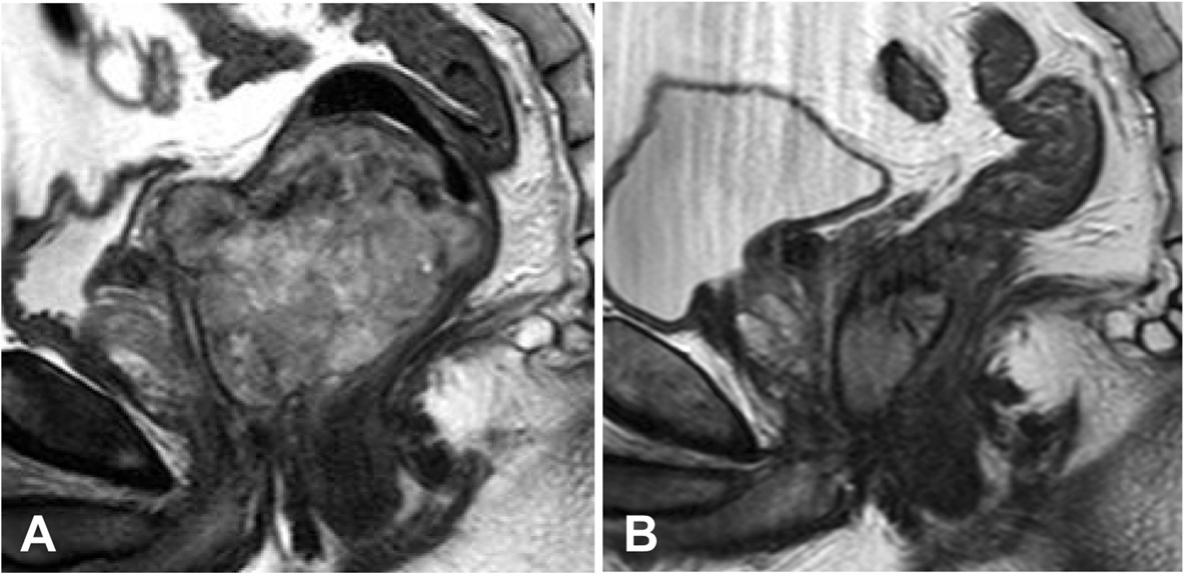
Sagittal view of pelvic MRI. a Before imatinib treatment, an 80-mm tumor was observed on the anterior wall of the lower rectum. b After 6 months treatment with imatinib, the tumor decreased to 37 mm
